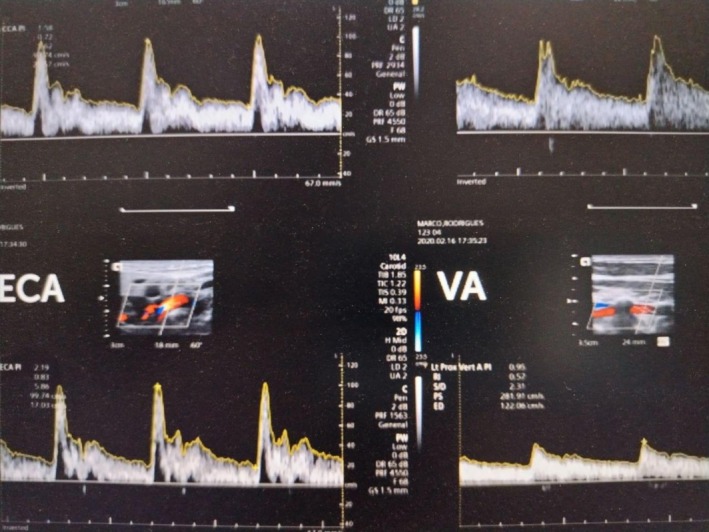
Carotid doppler study of a 52‐year‐old male with meningitis complicated with ischemic stroke and failed thrombolysis showing—Normal carotid artery doppler.
